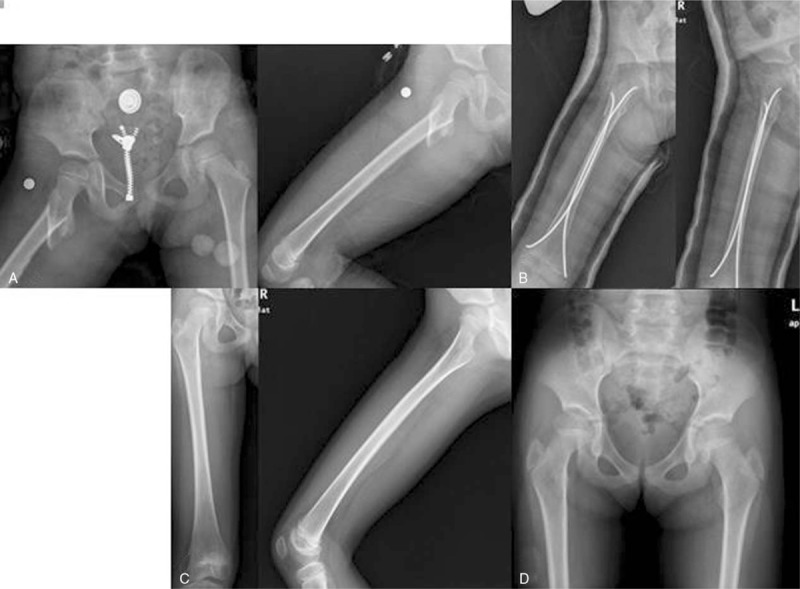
A 7.6-year-old female suffered traffic accident. (A) Preoperative radiographs of the right femur with short oblique stable subtrochanteric fracture. (B) Immediate postoperative radiographs after closed reduction and fixation with 2 nails. (C) The bone healed well 6 months after surgery. (D) The patient showed a normal NSA (as on the uninvolved side) and a 1.5 cm limb length discrepancy with overgrowth of involved side.
